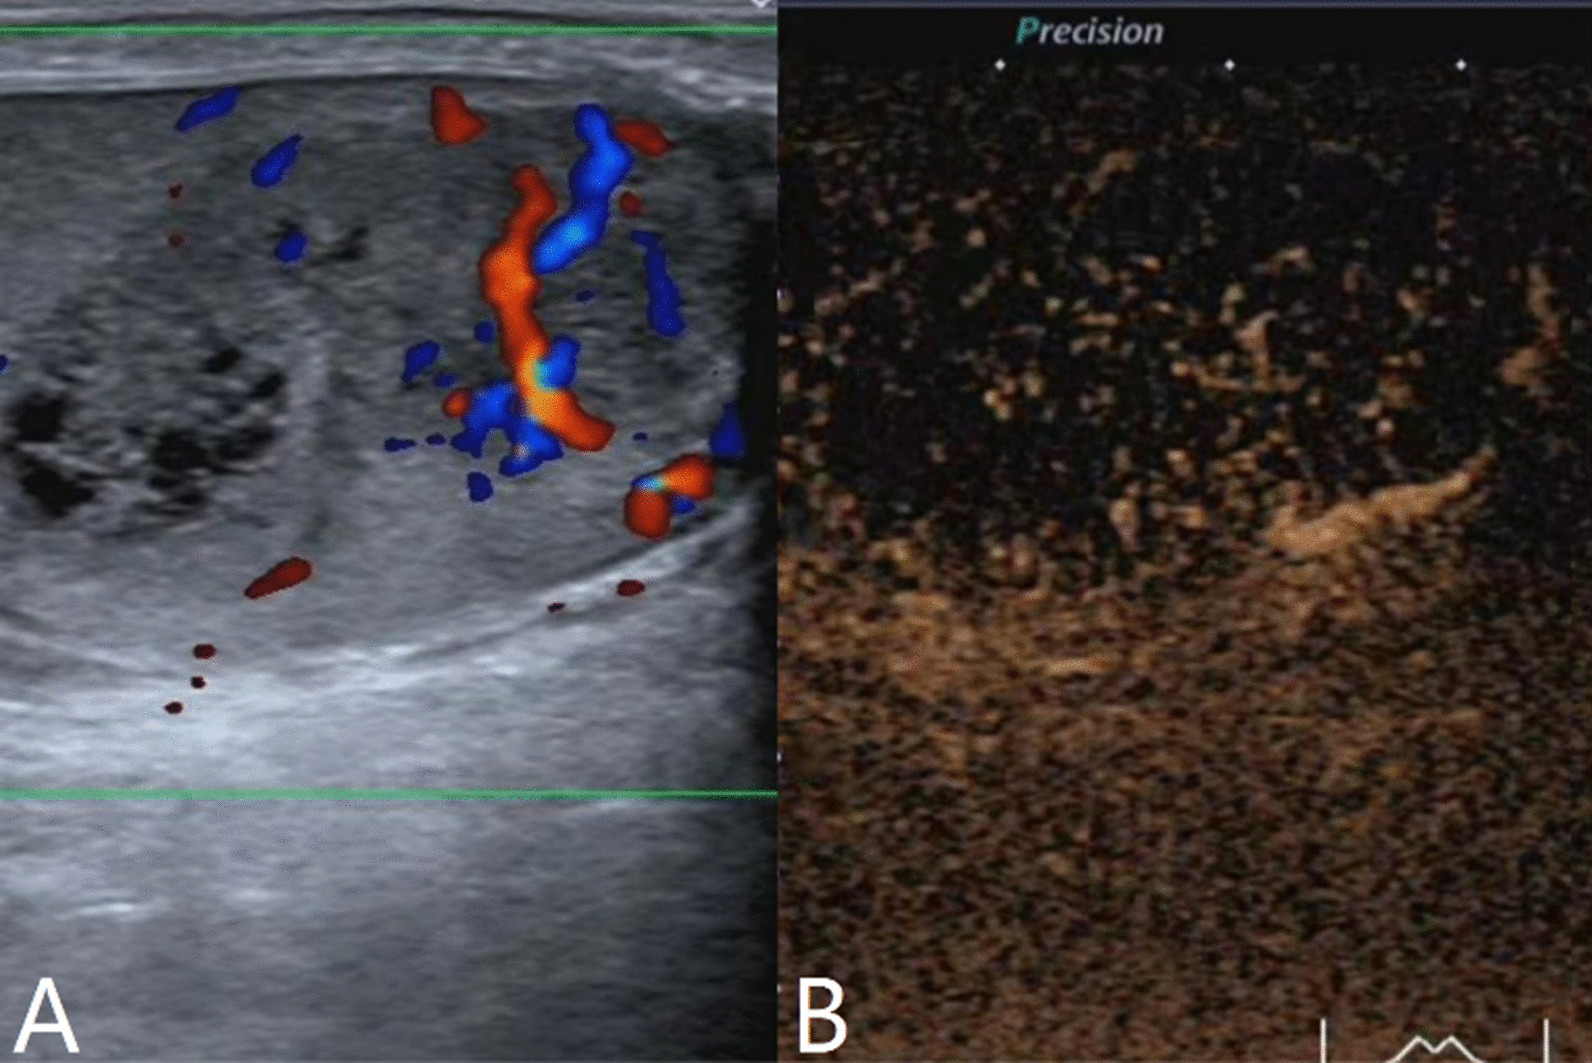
Testicular seminoma. A Conventional ultrasonography showed cystic and solid mixed echoes with clear borders in the right testis; the solid part was rich in blood flow. B Contrast-enhanced ultrasonography showed sparse and low enhancement
